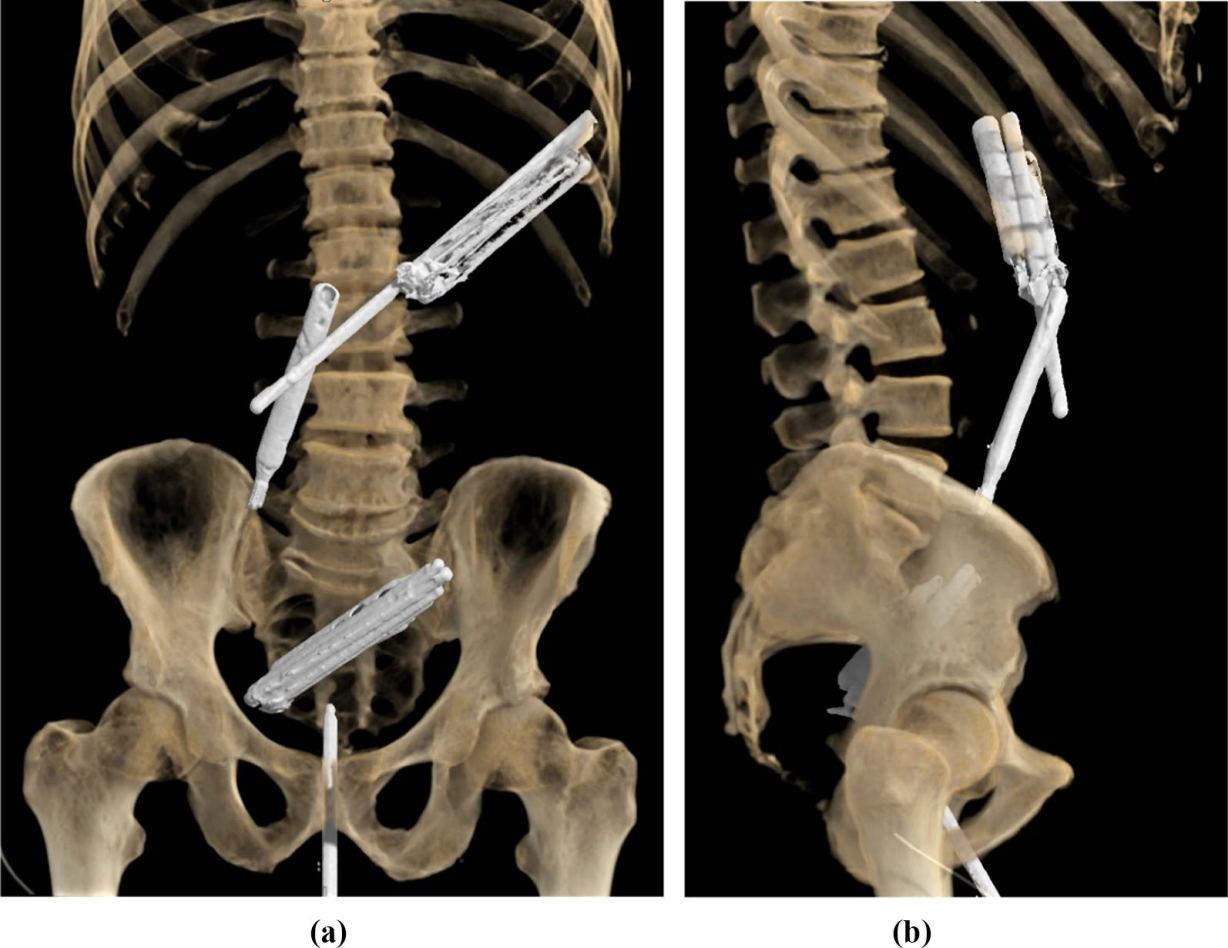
a, b 3D-reconstruction of CT scan
